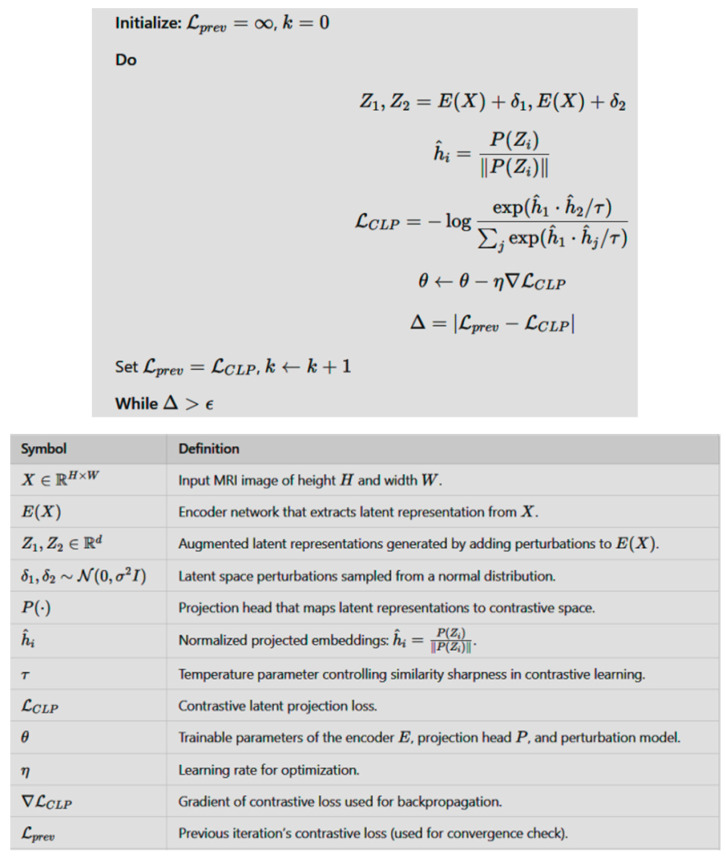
The do while code of CLP with the input MRI image (X), encoder, and projection head along with a description of the involved parameters.
